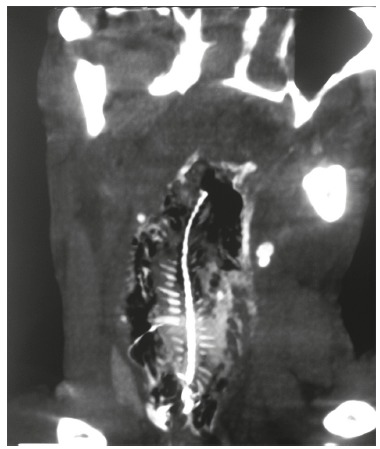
Coronal computed tomography of the neck clearly showing the fish impacted in cervical esophagus
